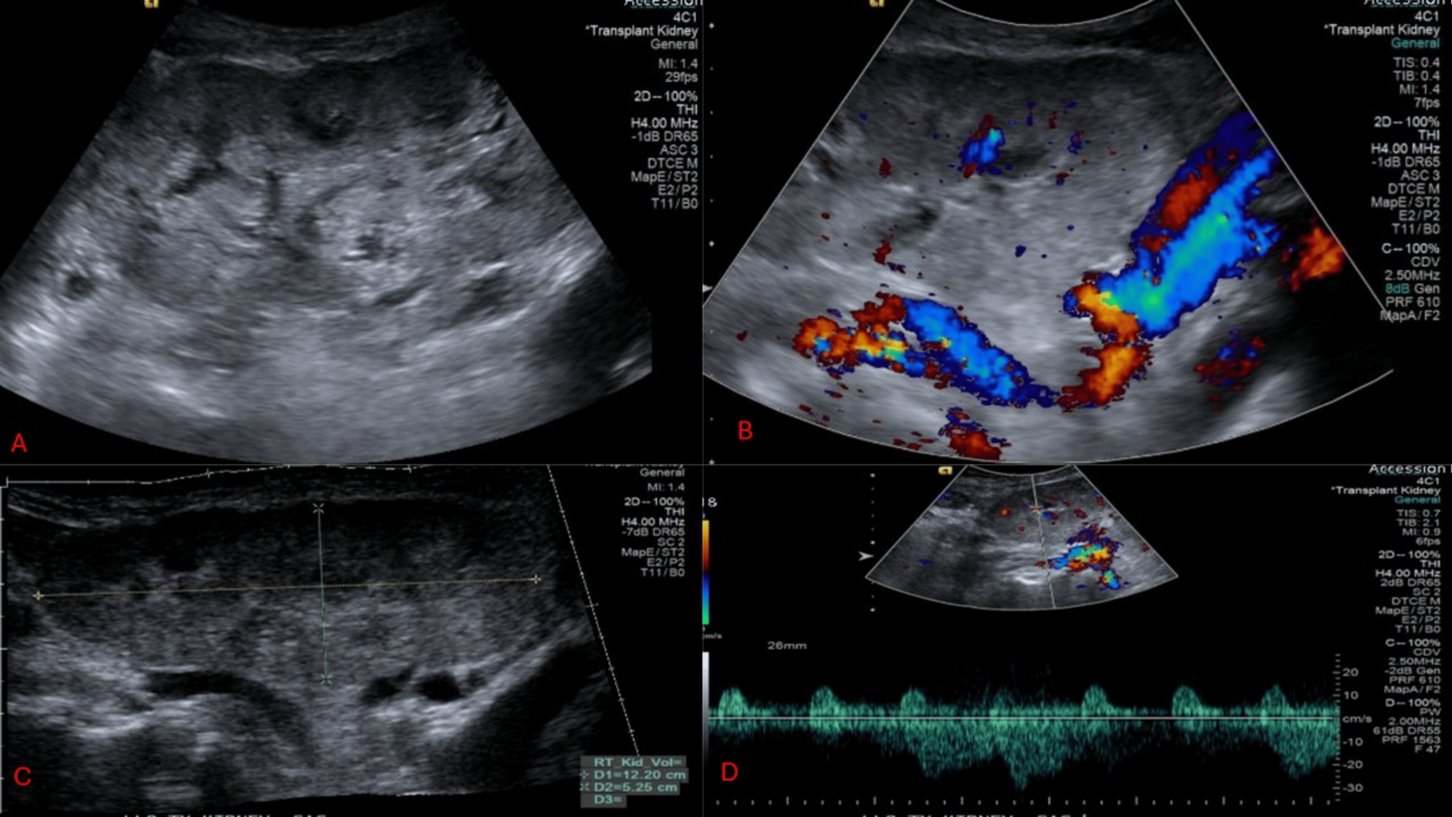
Transplanted kidney ultrasound (A) There is increased echogenicity, but normal parenchymal thickness and contour, no calculi, no pelvicalyceal dilation, no cysts, and no solid masses, with no peri-transplant fluid collection; (B) Doppler ultrasound of transplanted kidney; (C) Transplanted kidney size: 12.2 x 5.3 x 5.9 cm; (D) Transplanted kidney resistive indices: 0.64, 0.61, 0.60 at the upper, middle, and lower poles, respectively (normally less than 0.7).
